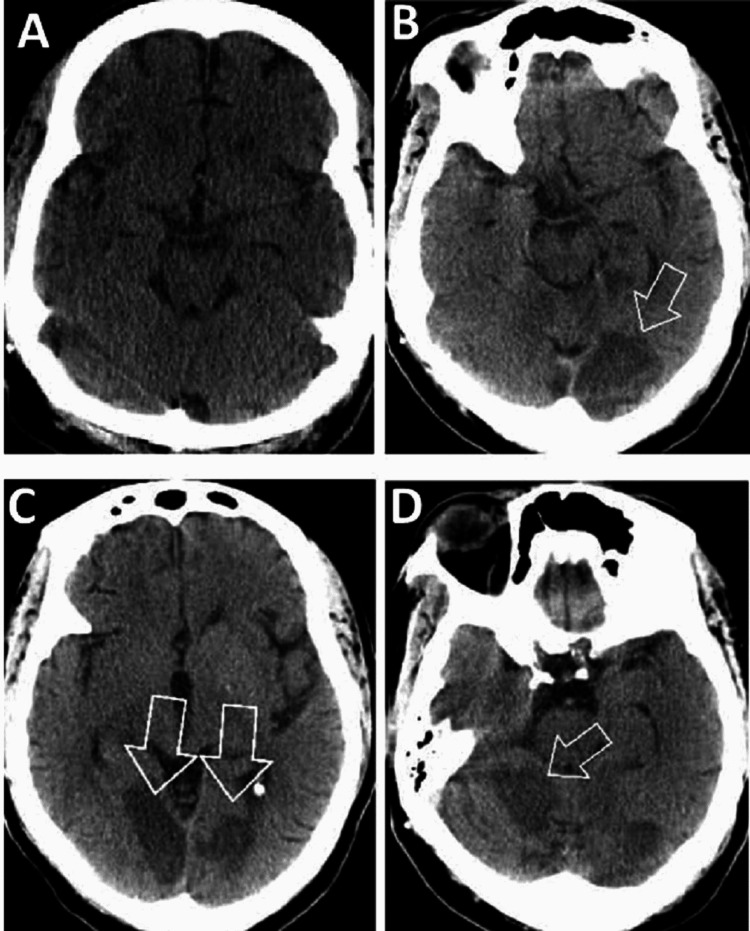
CT of the head without contrast at the time of admission and four days after admission A: CT of the head without contrast at the time of admission did not show any acute or subacute ischemic changes. B, C, and D: CT scans of the head without contrast four days after admission showed multifocal infarctions in the bilateral cerebellum, occipital lobe, and posterior left temporal lobe (arrowheads indicate infarctions).
